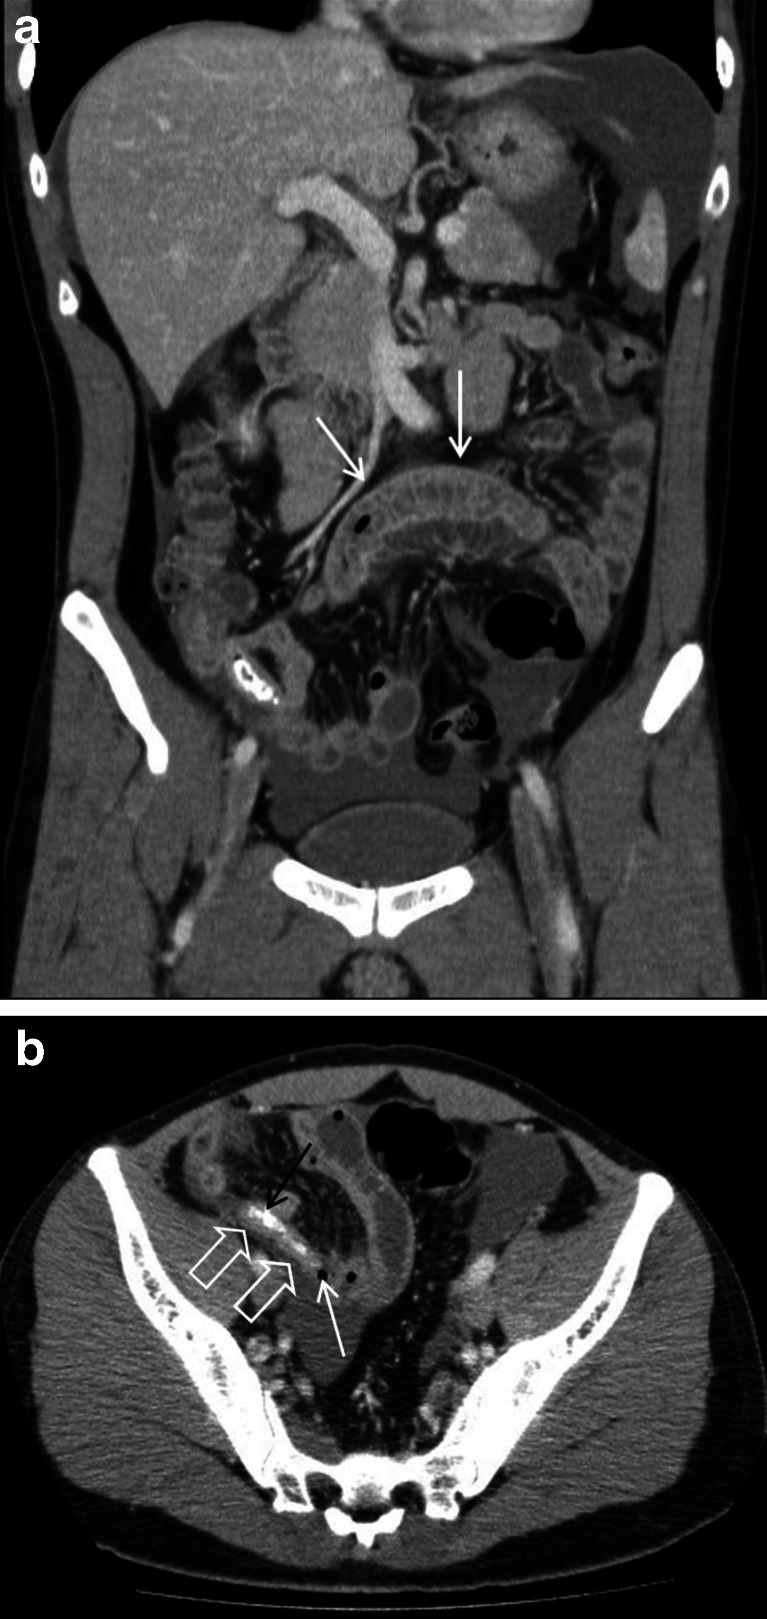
a A 32-year-old male with enteritis masquerading as acute appendicitis (case 2; see b). Contrast-enhanced coronal images demonstrate a fluid-filled small bowel loop with mural thickening and enhancement in keeping with enteritis (*white arrows*). Ascites is noted within the perisplenic region and pelvis. b A 32-year-old male with enteritis (case 2; see b). Contrast-enhanced axial CT image demonstrates a small air locule (*white arrow*) at the tip of a blind-ending tubular structure (*open white arrows*) within the right iliac fossa with a slightly thickened and enhancing wall, suggesting possible perforation of an acutely inflamed appendix. Intraluminal dense material was in keeping with an appendicolith (*black arrow*)
